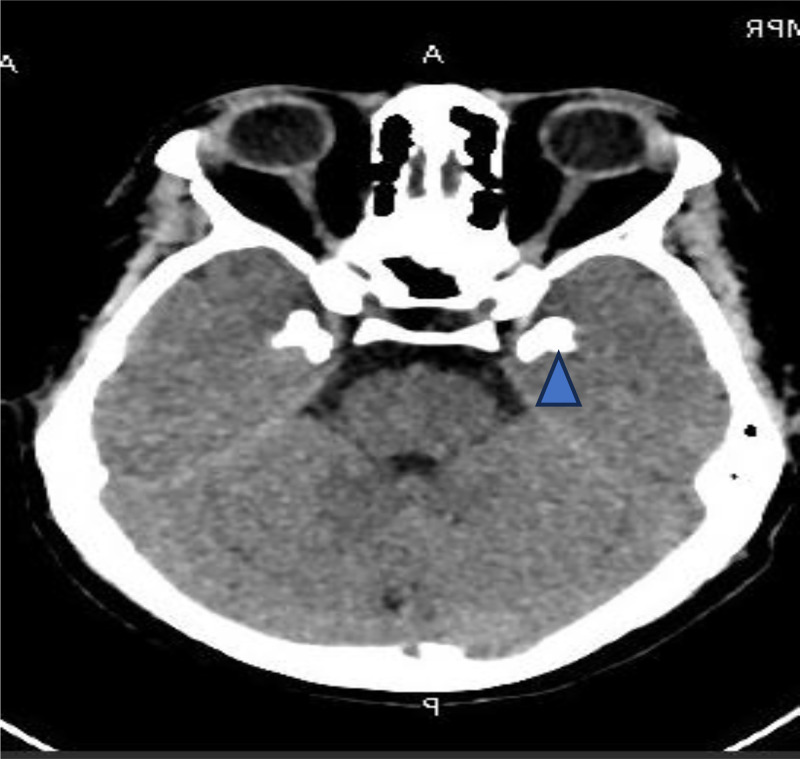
CT scan of the head showing the bilateral symmetrical dense calcifications.
